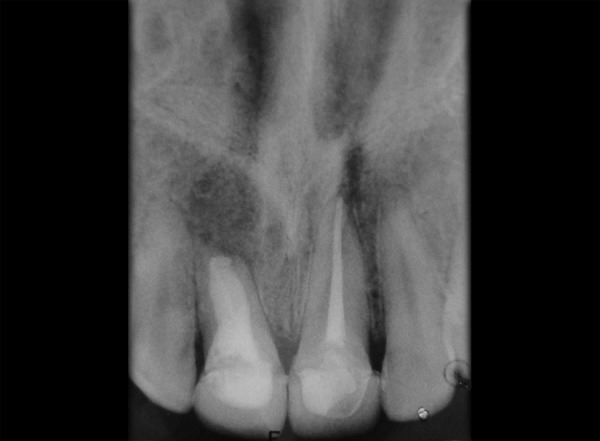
A 1-year and 6 months follow-up radiograph
